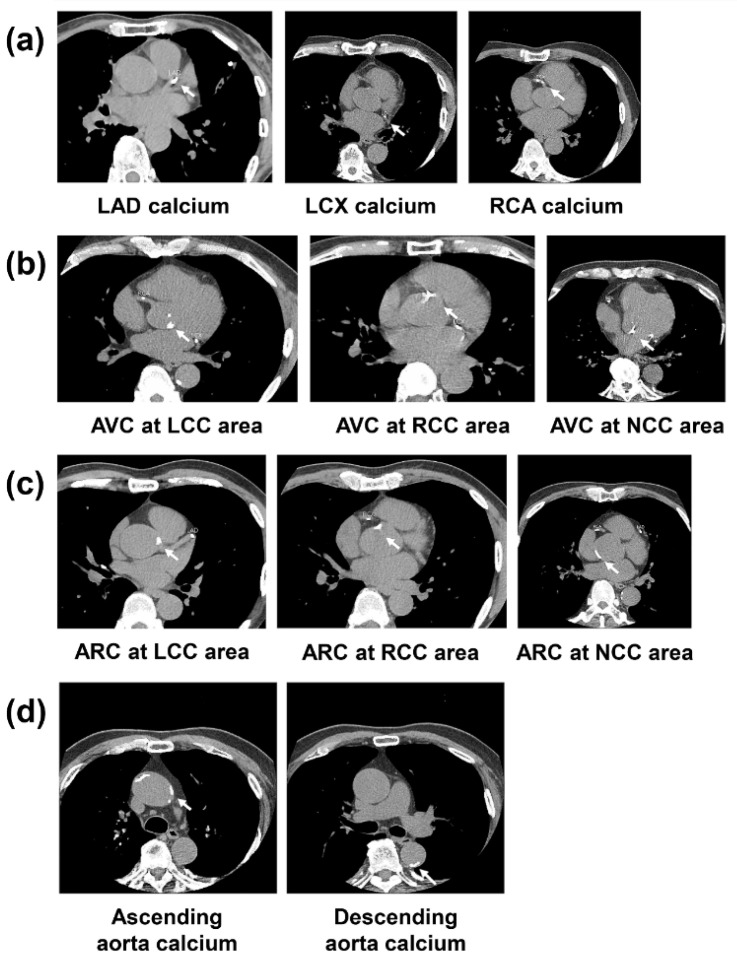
Cross-sectional cardiac CT of coronary artery calcium (a), aortic valve calcium (b), aortic root calcium (c), and thoracic aorta calcium (d). Abbreviations: ARC = aortic root calcium; AVC = aortic valve calcium; LAD = left anterior descending; LCC = left coronary cusp; LCX = left circumflex; NCC = noncoronary cusp; RCA = right coronary artery; RCC = right coronary cusp.
